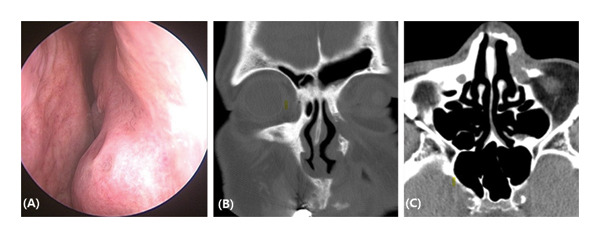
Postoperative 18‐month endoscopic finding (A), coronal (B), and axial (C) CT scans revealed no evidence of recurrence and facial deformity.
